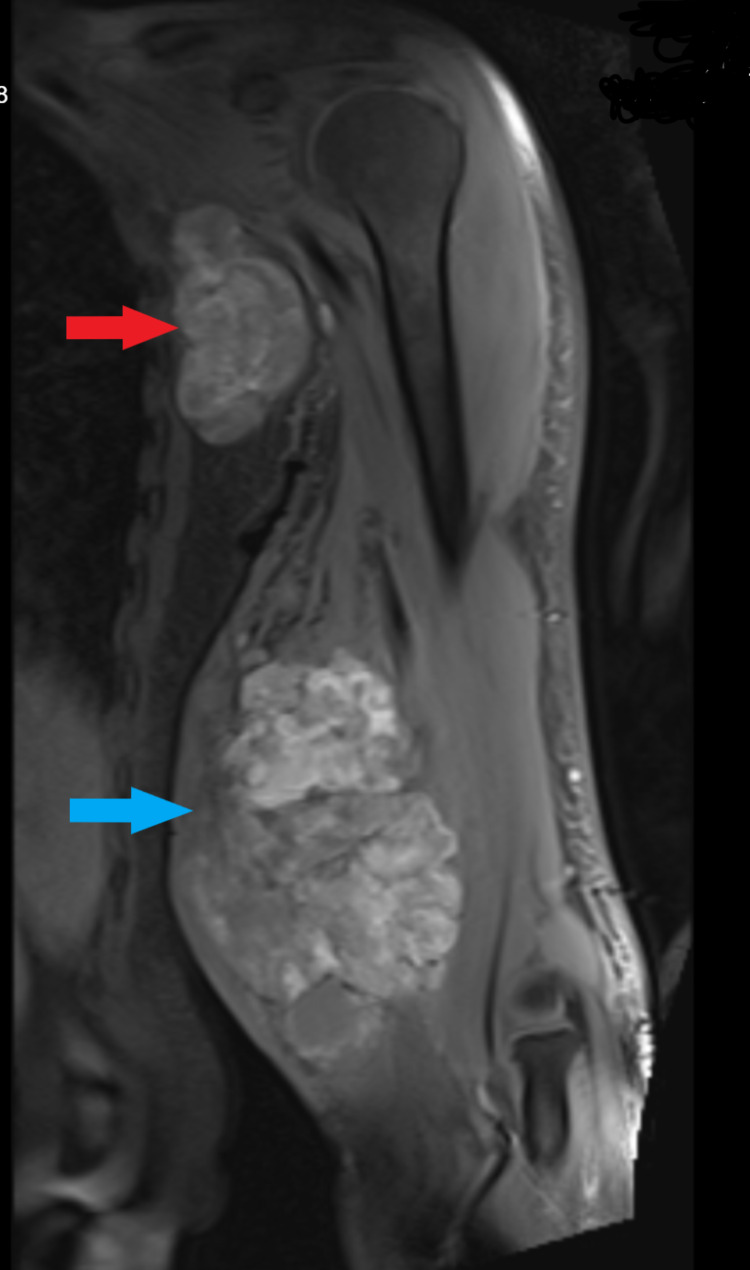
MRI of the arm showed heterogenous mass on the medial aspect of the left arm (blue arrow) and matted axillary lymph nodes (red arrow).
